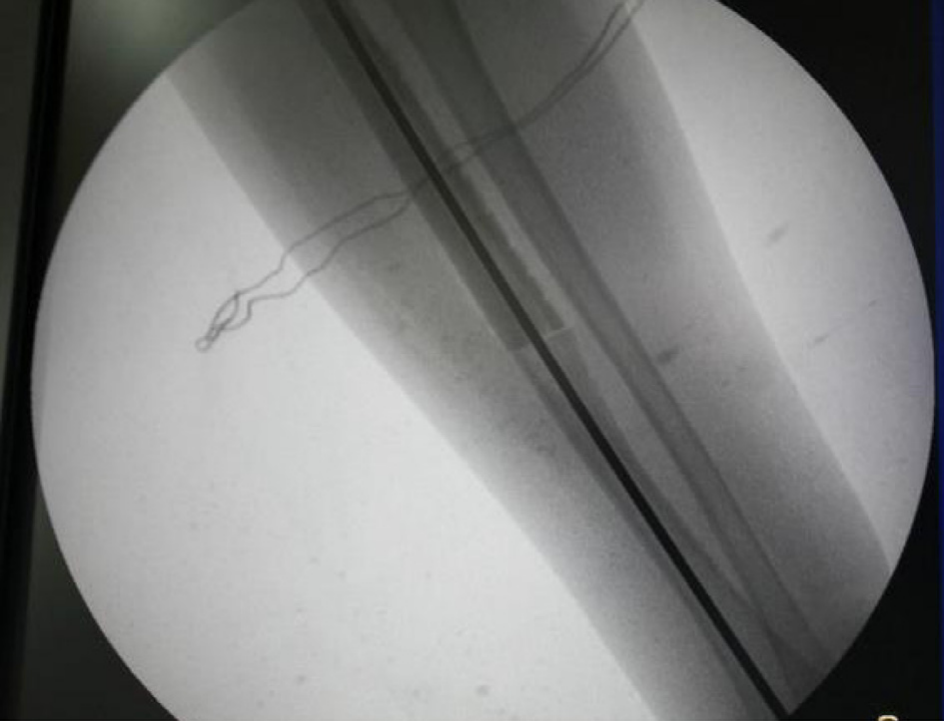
Intraoperative roentgenograph showing reconstruction of the radius using maternal fibular graft fixed with an intramedullary K-wire.
